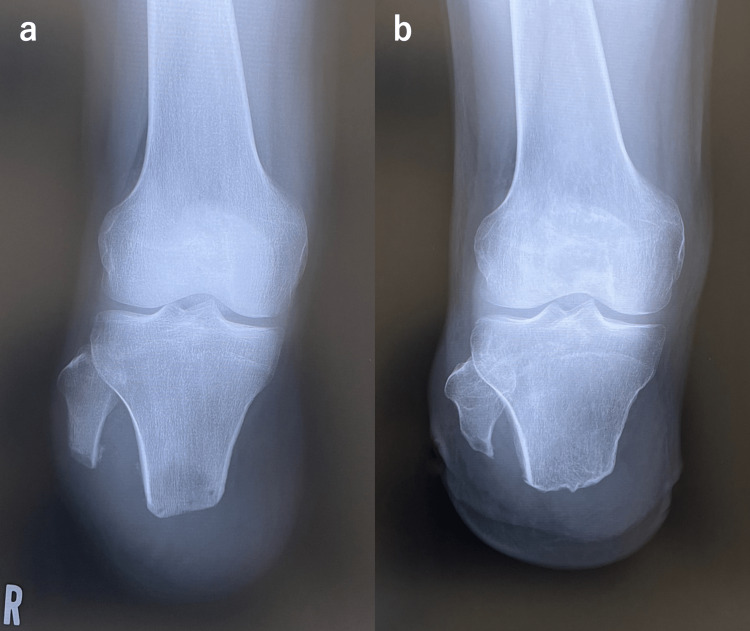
Plain radiographs of the stump in Case 2. (a) One month and a half after surgery. The tibial length is 8.9 cm. (b) Seventy-four months after surgery. The tibial length is 7.5 cm. The tibial length was measured straight from the medial tibial plateau to the distal end.
